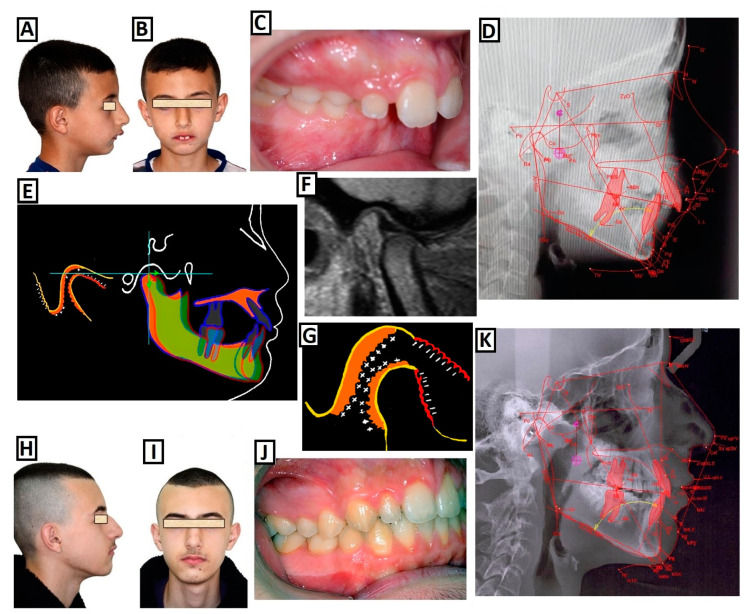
Treatment of class II dysgnathy during the growing ages through growth-influencing methods. (A–D) Before treatment: (A) lateral view photo of the patient, (B) frontal view photo of the patient, (C) intraoral photo, and (D) cephalometric radiograph. (E–G) The use of the device to influence growth: creating new conditions for the jaw condyles and, thus, intensifying growth in the fossa and condyles. Mandibular advancement through the construction bite causes bone resorption and bone apposition in the fossa and condyles. The MRI image shows the new position of the condyles with the construction bite. (H–K) After treatment: (H) lateral view photo of the patient, (I) frontal view photo, (J) intraoral photo, and (K) cephalometric radiograph.
